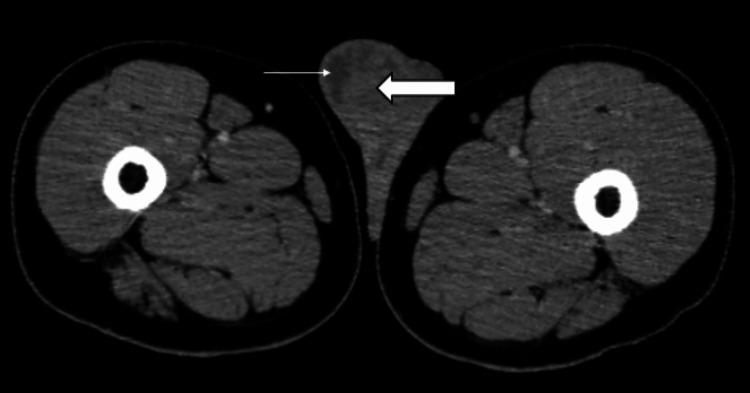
Post-contrast axial CT section at the level of testis showing heterogeneously enhancing right testicular lesion (block arrow) with cystic areas noted within (line arrow).
